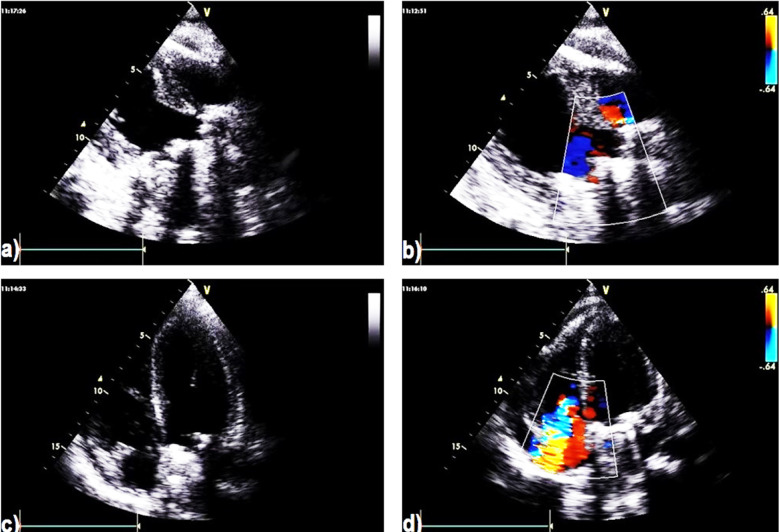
Transthoracic echocardiography (TTE) post-operative images show a) aortic valve replacement, b) Color flow mapping (CFM) showing good functioning metallic aortic and mitral valves, c) apical view showing good positioning of the mitral valve, d) Color flow mapping (CFM) revealing remnant mild tricuspid regurgitation (TR).
